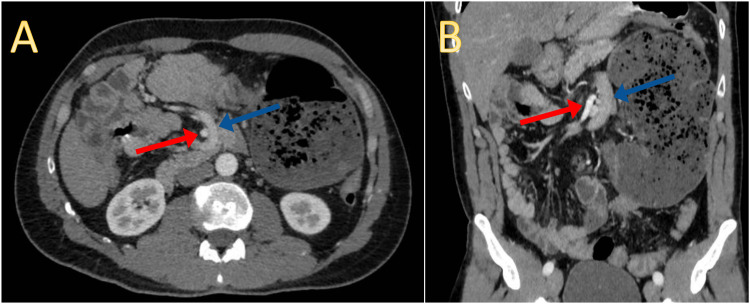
Abdominal contrast enhanced CT in axial (A) and coronal (B) views showing reversed relationship of the SMA (red arrow) and SMV (blue arrow), a pathognomonic sign of intestinal nonrotation.
